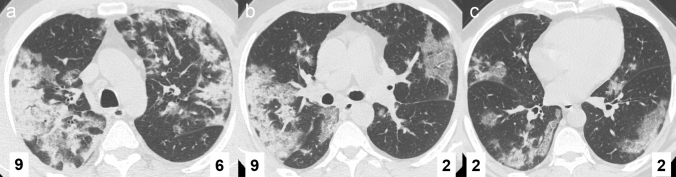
HRCT scans from the upper (a), middle (b), and lower (c) zones in a 54-year-old man who developed a severe course of COVID-19 pneumonia. The total CT severity score is 30, based on the scoring system proposed by Yuan et al. [64]: a right upper, 3 (consolidation) × 3 (extent 50–75%); left upper, 3 (consolidation) × 2 (25–50%); b right middle, 3 (consolidation) × 3 (50–75%); left middle, 2 (ground-glass) × 1 (< 25%); c right lower, 2 (ground-glass) × 1 (< 25%); left lower, 2 (ground-glass) × 1 (< 25%)
